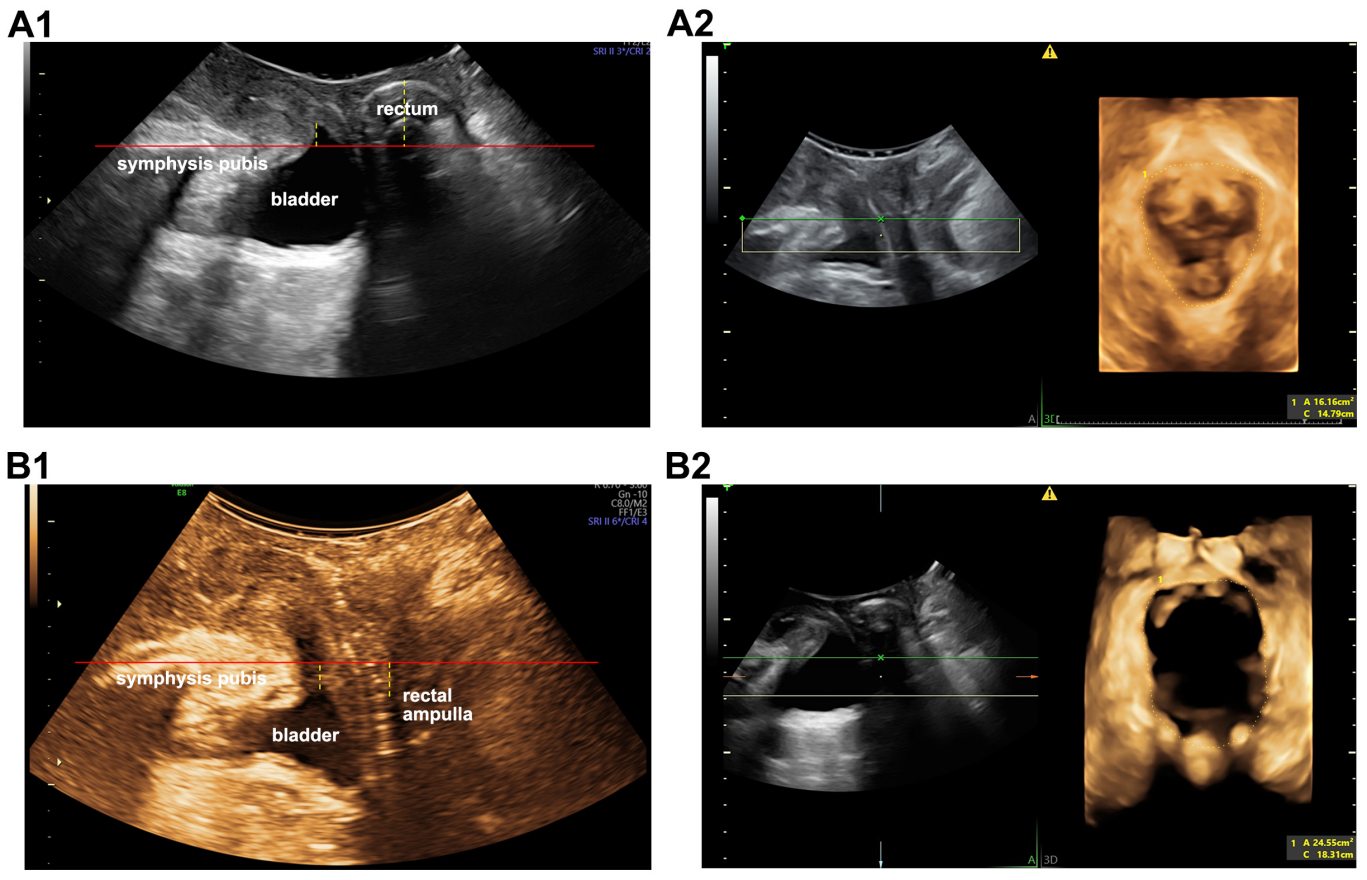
Ultrasound images of the patient with constipation before and after 7 years. (A1) Images in 2015 were without any positive features. (A2) The levator hiatus area in 2015 year was 16.16 cm^2^. (B1) Images in the 2022 year were diagnosed as vesicocele and rectocele. (B2) The levator hiatus area was 24.55 cm^2^.
